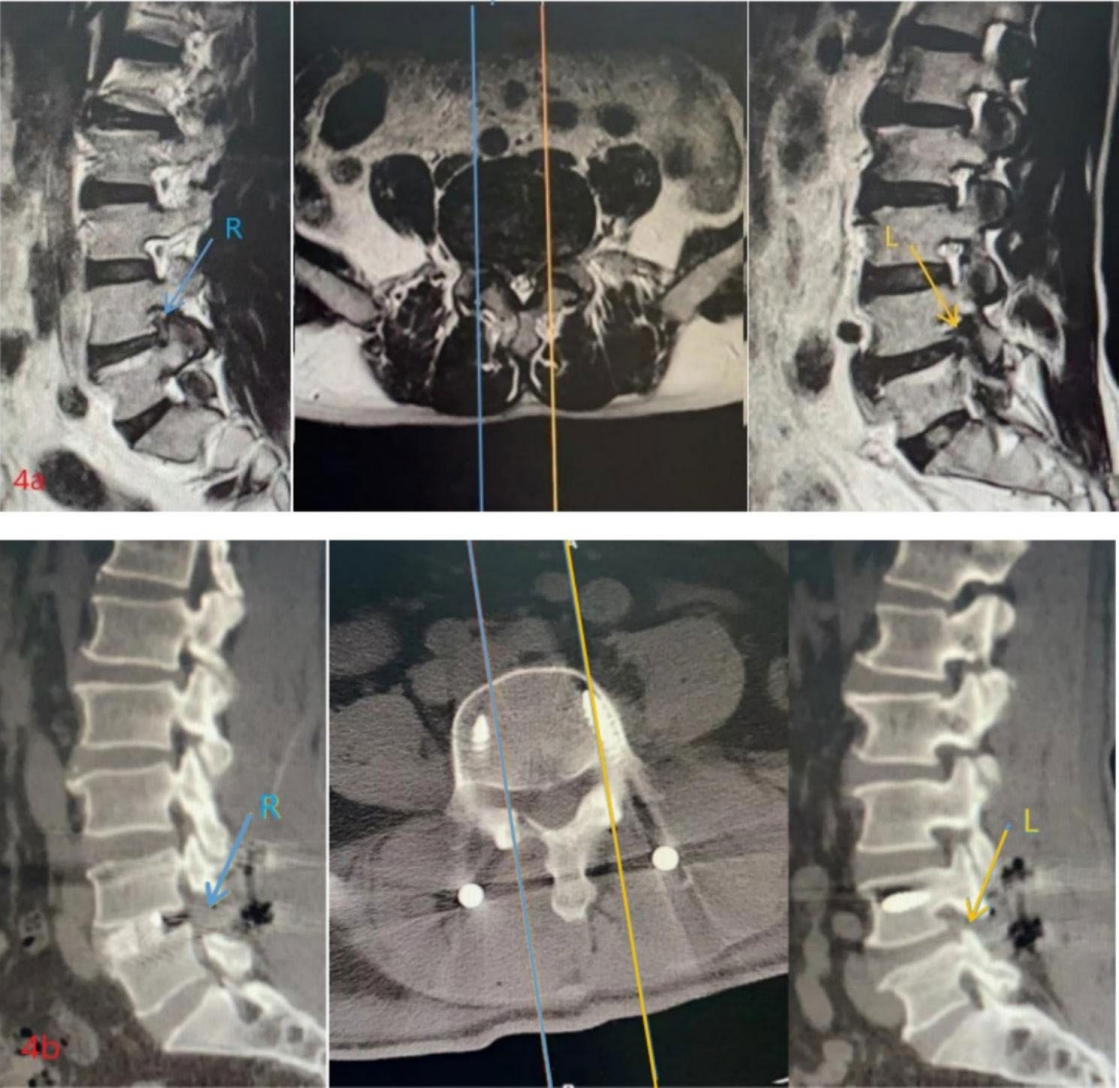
A 60-year-old male patient with low back pain and right lower limb pain and numbness was hospitalized for one month. Lumbar decompression and interbody fusion was performed through the right intervertebral foramen of L4/5. The cortical bone of L4/5 left facet joint and transverse process were removed during operation. during the operation, and preventive decompression was performed. The operation was successful, the postoperative recovery was good, and no contralateral root symptoms occurred. 4a, Preoperative MRI showed severe stenosis of the L4/5 bilateral intervertebral foramen. 4b, The right lamina and facet joint of L4/5 were removed. The cortical bone of L4/5 left facet joint and transverse process were removed
